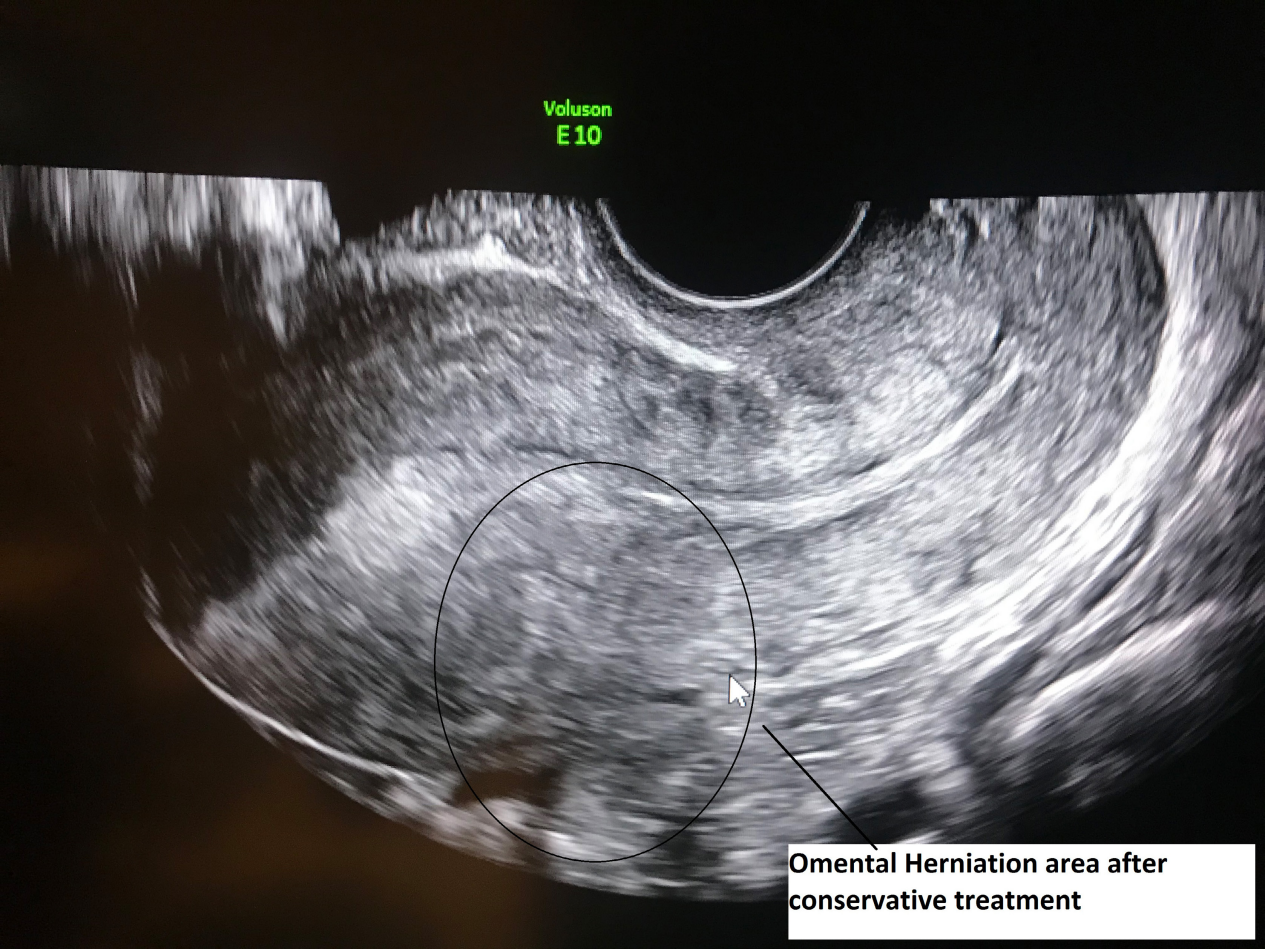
Transvaginal ultrasonographic image of the case after conservative approach.
